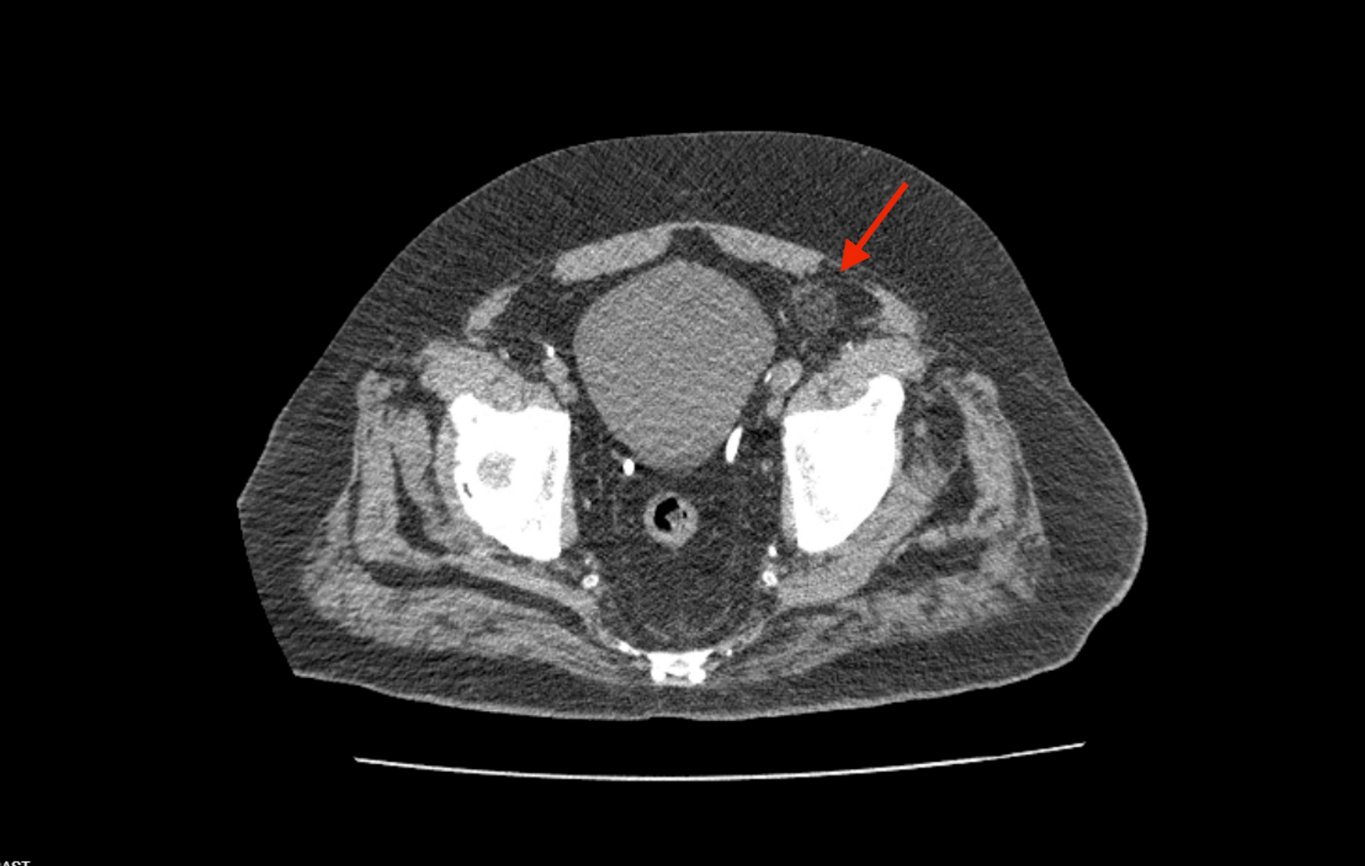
CT abdomen and pelvis demonstrating sigmoid epiploic appendagitis CT: computed tomography
